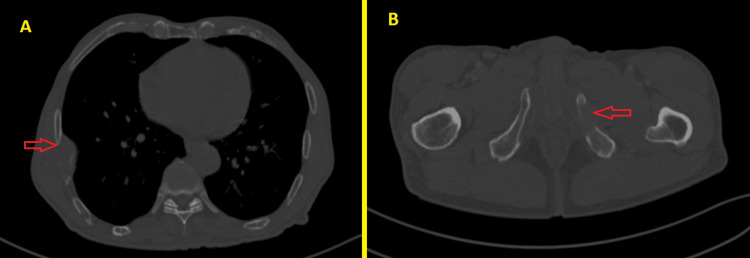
Thoracic-abdomen-pelvis CT scan showing the right rib (A) and left pubic infiltration (B) (red arrows).
